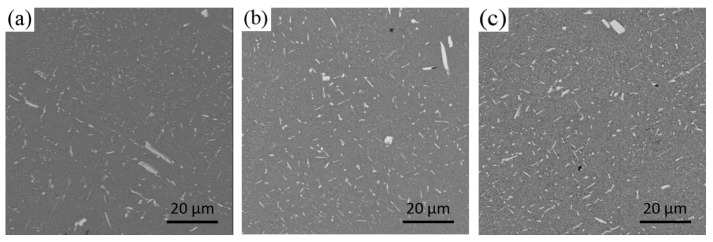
Distribution of the secondary phase in the grain at the 1/2 radius: (a) No ultrasonic treatment; (b) treatment by the L-shaped ultrasonic wave guide rod; (c) treatment by the straight-rod ultrasonic wave guide rod.
